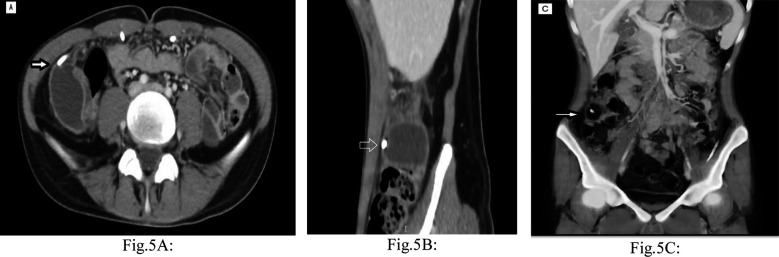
Contrast-enhanced CT abdomen scan (A) axial, (B) sagittal, and (C) coronal 3D reconstruction images demonstrate APC in the right iliac fossa with the tip of the right-sided VPS peritoneal catheter barely inside it (white arrows).
